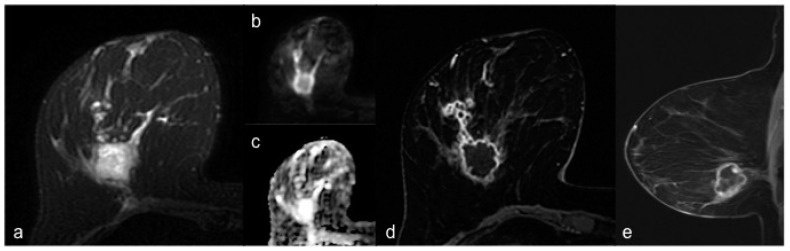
Breast MRI of a 53-year-old woman with TNBC of the right breast. (a) Axial fat-suppressed T2-weighted image shows a hyperintense round mass with irregular margins in the lower outer quadrant of the right breast. (b) Axial DWI image (b value = 1000 s/mm^2^) shows peripheral high signal intensity and central hypointensity. The mass appears homogeneously hyperintense in the corresponding ADC map (c). (d) Axial and sagittal (e) post-contrast T1-weighted images show a corresponding irregular round mass with rim enhancement and some small foci of enhancement connected posteriorly by thin striae.
